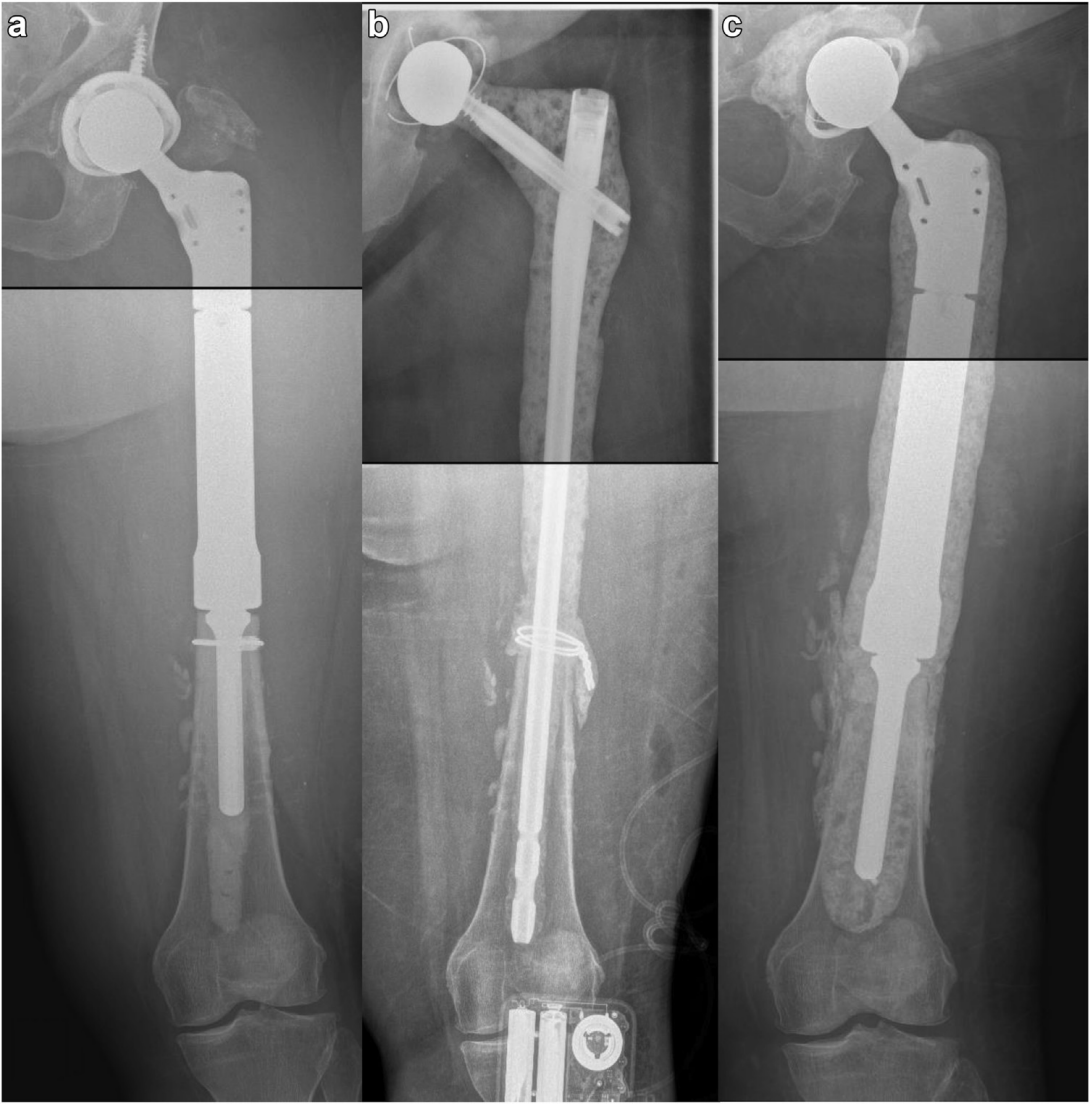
Case 1 RAH. (a) Anteroposterior (AP) radiographs demonstrating infected left proximal femur replacement. (b) AP radiographs after the first-stage reconstruction of the femur using an antibiotic-cement-coated gamma nail as an interval prosthesis. (c) AP radiographs of second-stage revision with an antibiotic-cement-coated proximal femur replacement prosthesis.
